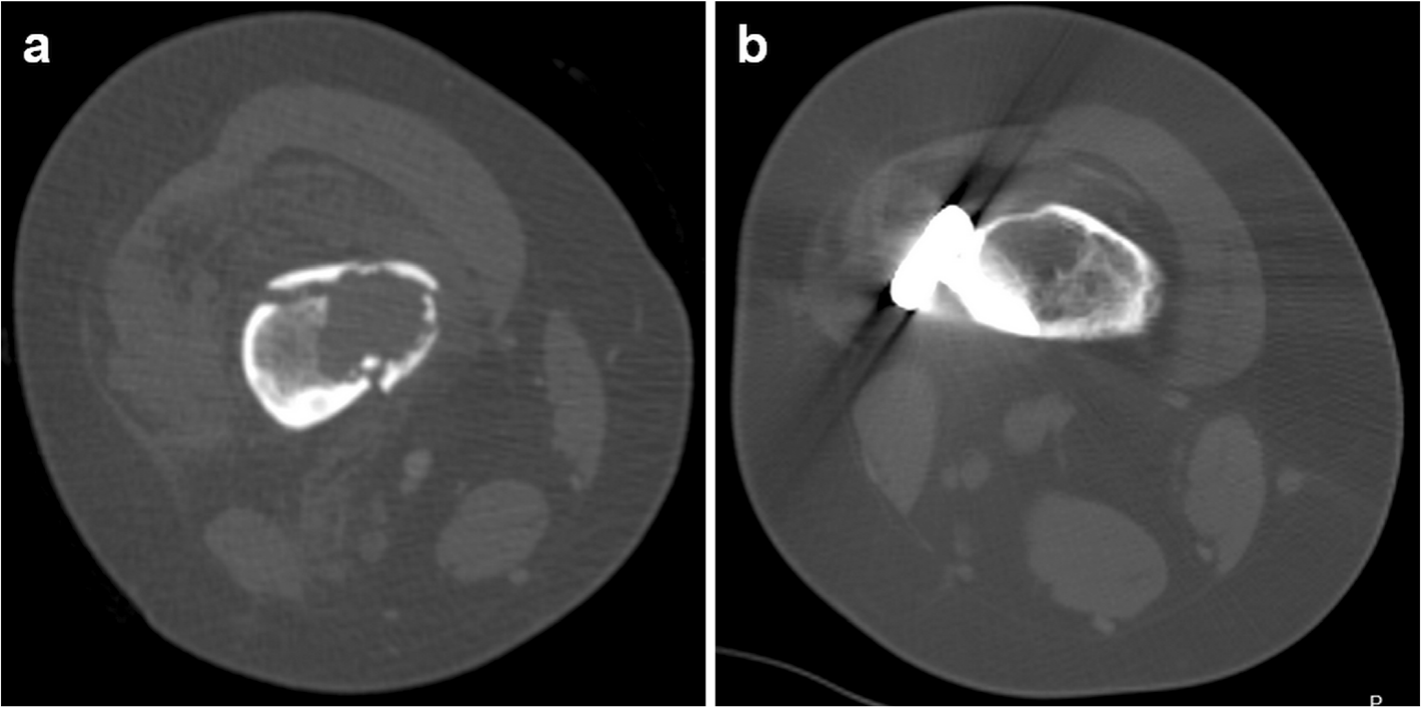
Pre-operative (a) and 18-month follow-up CT (b) demonstrating the healing of one biopsied NOF.
